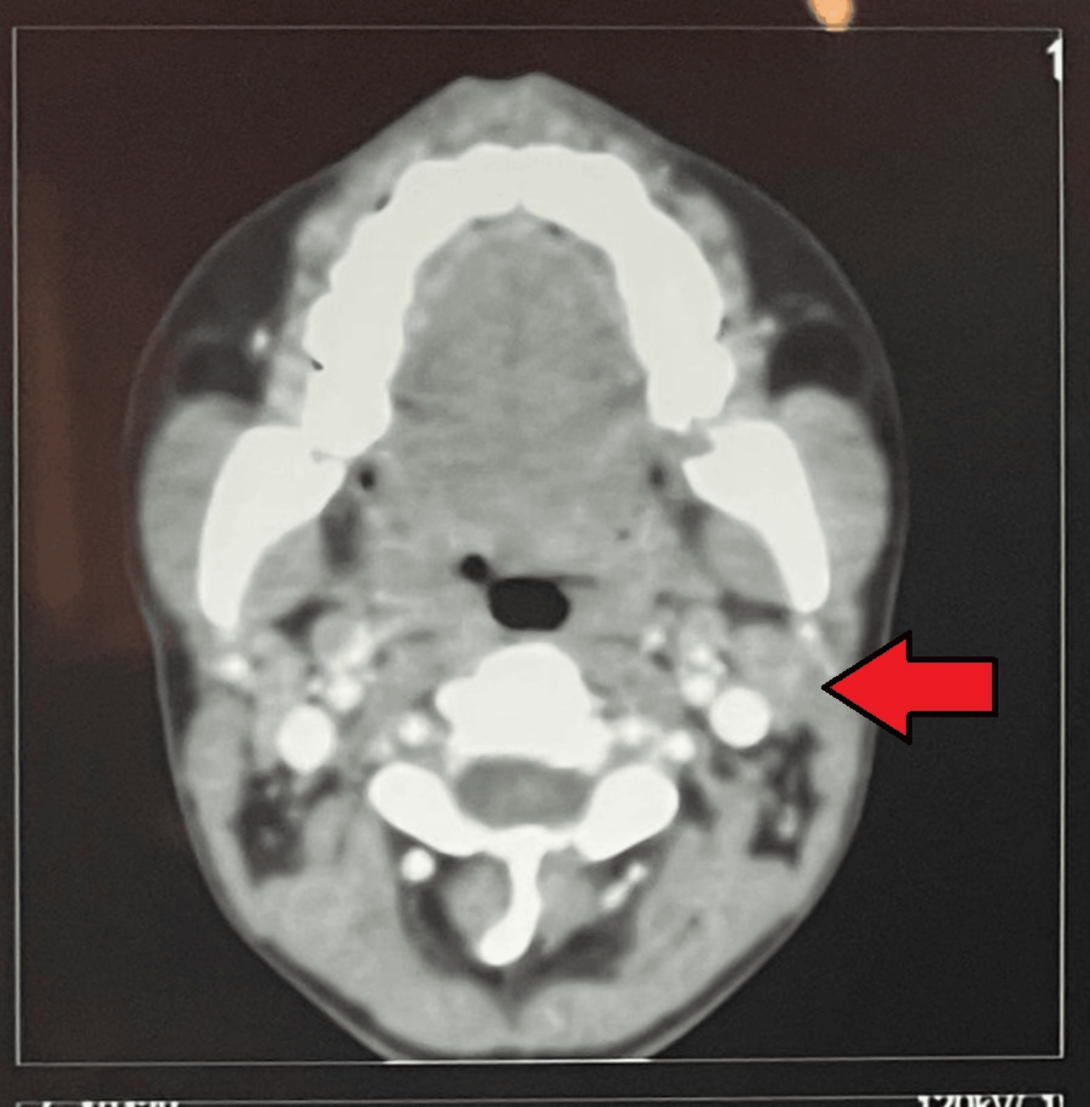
CT neck with contrast showing extensive lymphadenopathy, arrow points to enlarged lymph nodes, patient identifiers digitally concealed. CT: Computed axial tomographic scan.
